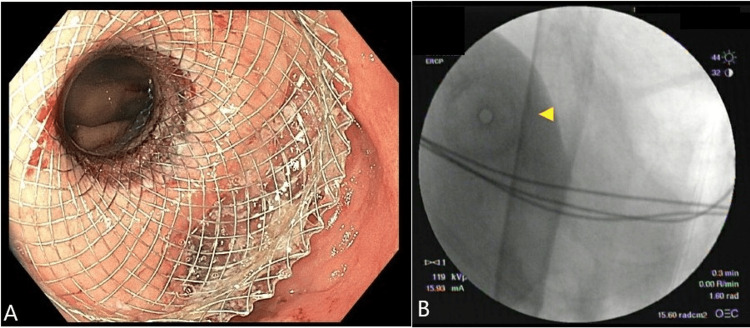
AXIOS Stent Shown in an Endoscopic Ultrasound Shown is an endoscopic ultrasound (EUS) demonstrating an AXIOS stent. Figure 1A shows the AXIOS stent traversing the Roux-en-Y. The arrow in Figure 1B indicates the location of the AXIOS stent, which was deployed between the gastric pouch and excluded stomach
